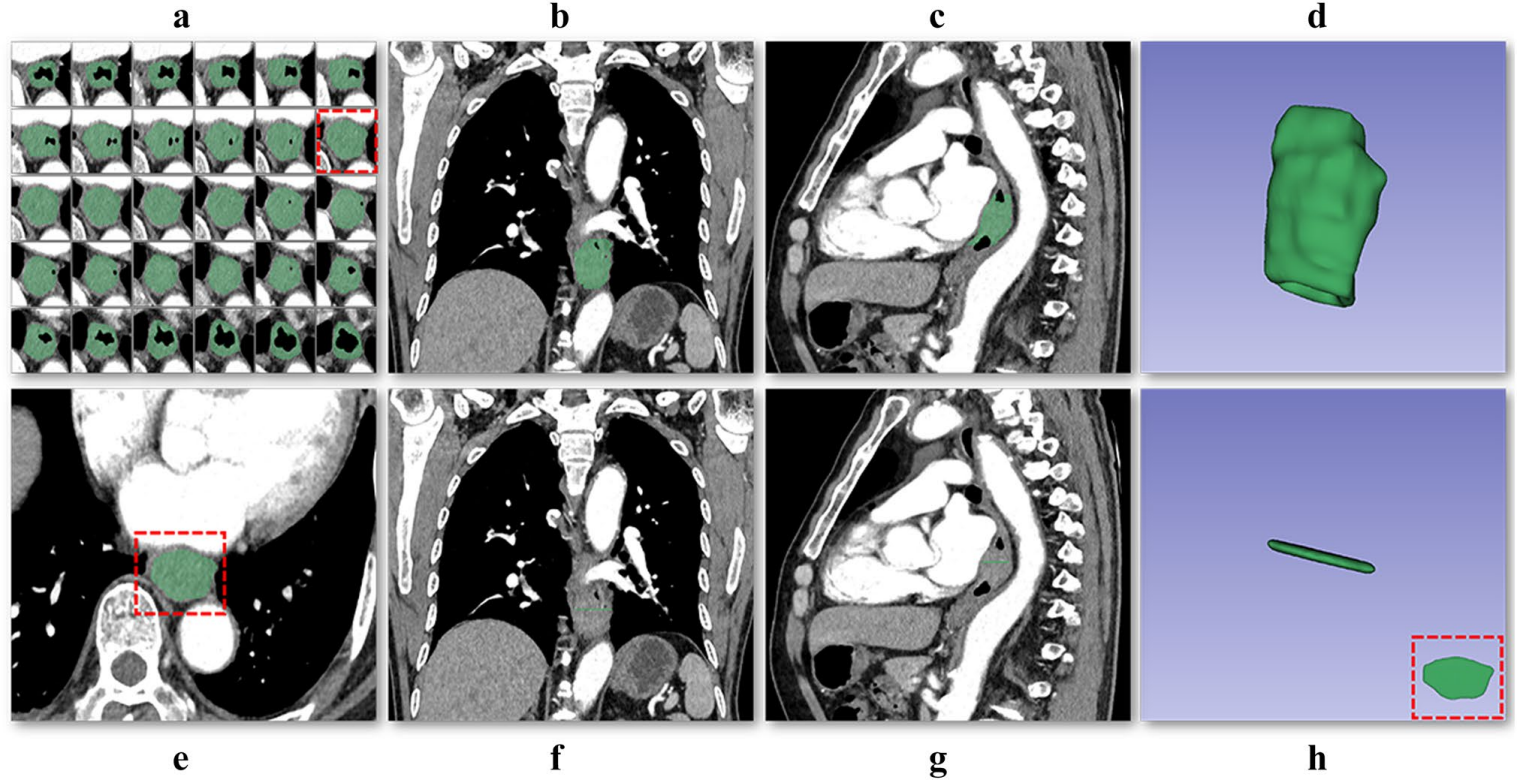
The process of tumor segmentation. The 3D ROI covered the whole tumor area by delineating the tumor tissue layer by layer on axial CT images (a). Subsequently, adjustments were made with reference to the coronal (b) and sagittal (c) CT images to finally reconstruct the 3D ROI (d). The 2D ROI was delineated on the largest cross-sectional area of the tumor (e). Subsequently, adjustments were made with reference to the coronal (f) and sagittal (g) CT images to finally reconstruct the 2D ROI (h)
